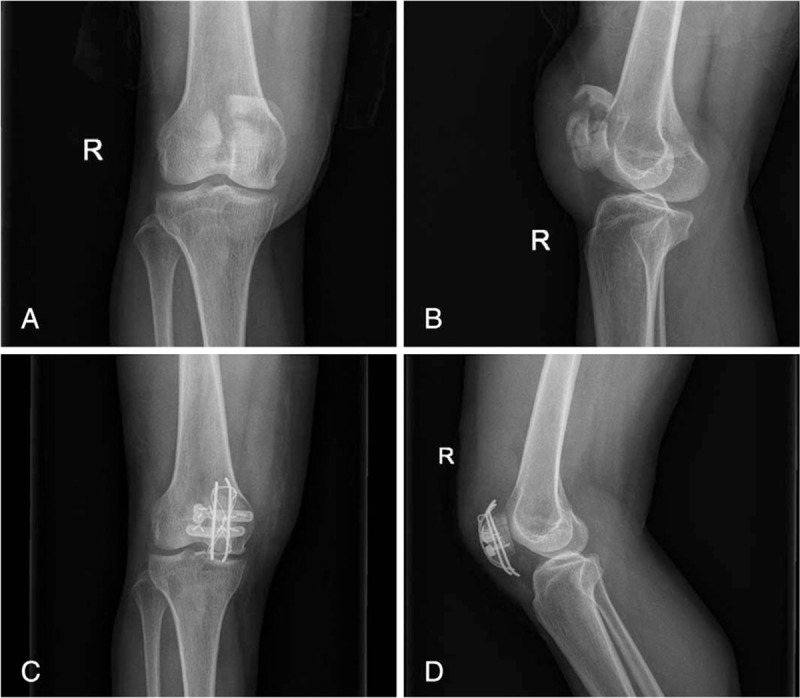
Radiographs of the comminuted patellar fracture (A and B). Radiographs after osteosynthesis (C and D).
